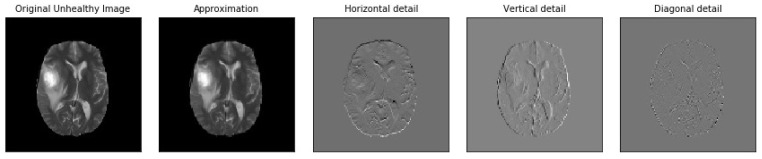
Samples of our Level 1 DWT decomposition of a non-healthy MR image type T2.
